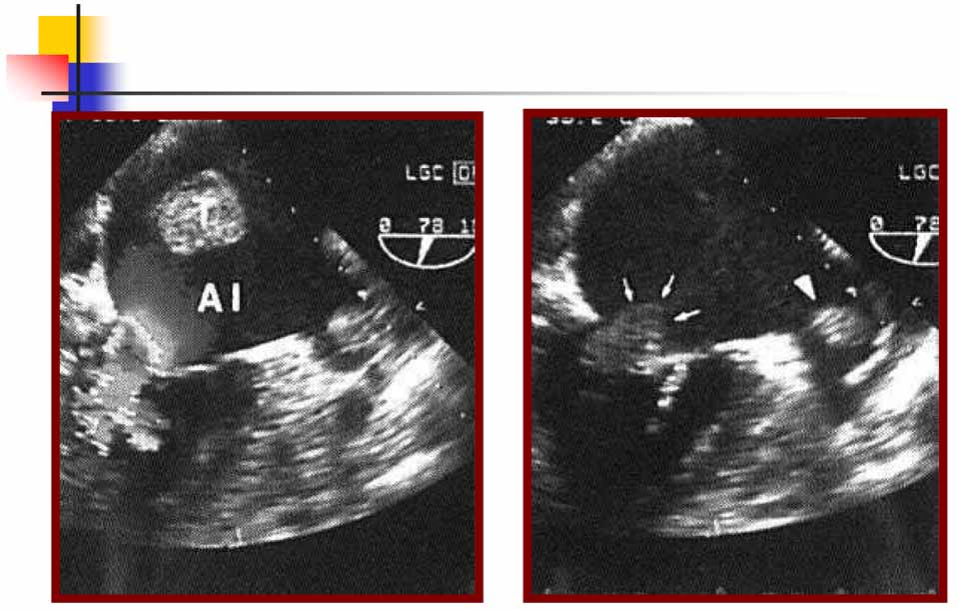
Transthoracic echocardiography reveals a thrombus in the left atrium (T) in a patient with double rheumatic mitral valve lesion and atrial fibrillation.
